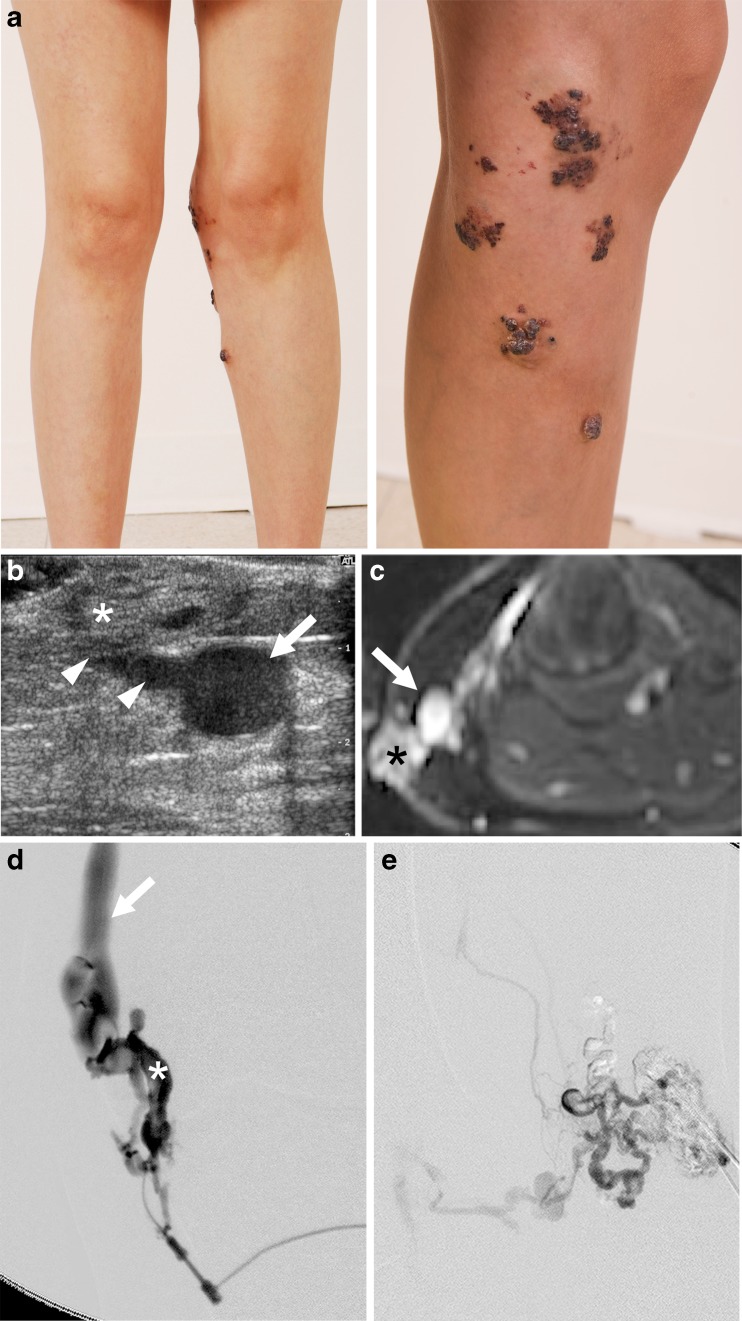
Multifocal venous malformations in a 16-year-old girl. a Clinical photographs demonstrate multifocal venous malformation located in skin and subcutaneous tissue at the knee. Venous malformations were also located in intramuscular tissues of the upper and lower thigh (not shown). b, c Ultrasound (b) and axial contrast-enhanced T1-weighted fat-saturated MRI (c) of subcutaneous soft tissues on the medial aspect of the knee show an ectatic greater saphenous vein *(arrow)* with connecting draining veins *(arrowheads)* from the subcutaneous component *(asterisk)* of the skin venous malformations. d Direct puncture venogram of subcutaneous venous malformation *(asterisk)* shows direct communication with the greater saphenous vein *(arrow)*. Endovenous laser ablation of the greater saphenous vein was performed. e Repeat venogram of subcutaneous venous malformation following endovenous laser ablation of the greater saphenous vein shows reduced communication with the greater saphenous vein, which allowed for sclerotherapy of the venous malformation. Following endovenous laser ablation and sclerotherapy, pain and medial knee swelling improved and selected skin venous malformations became flatter and paler
